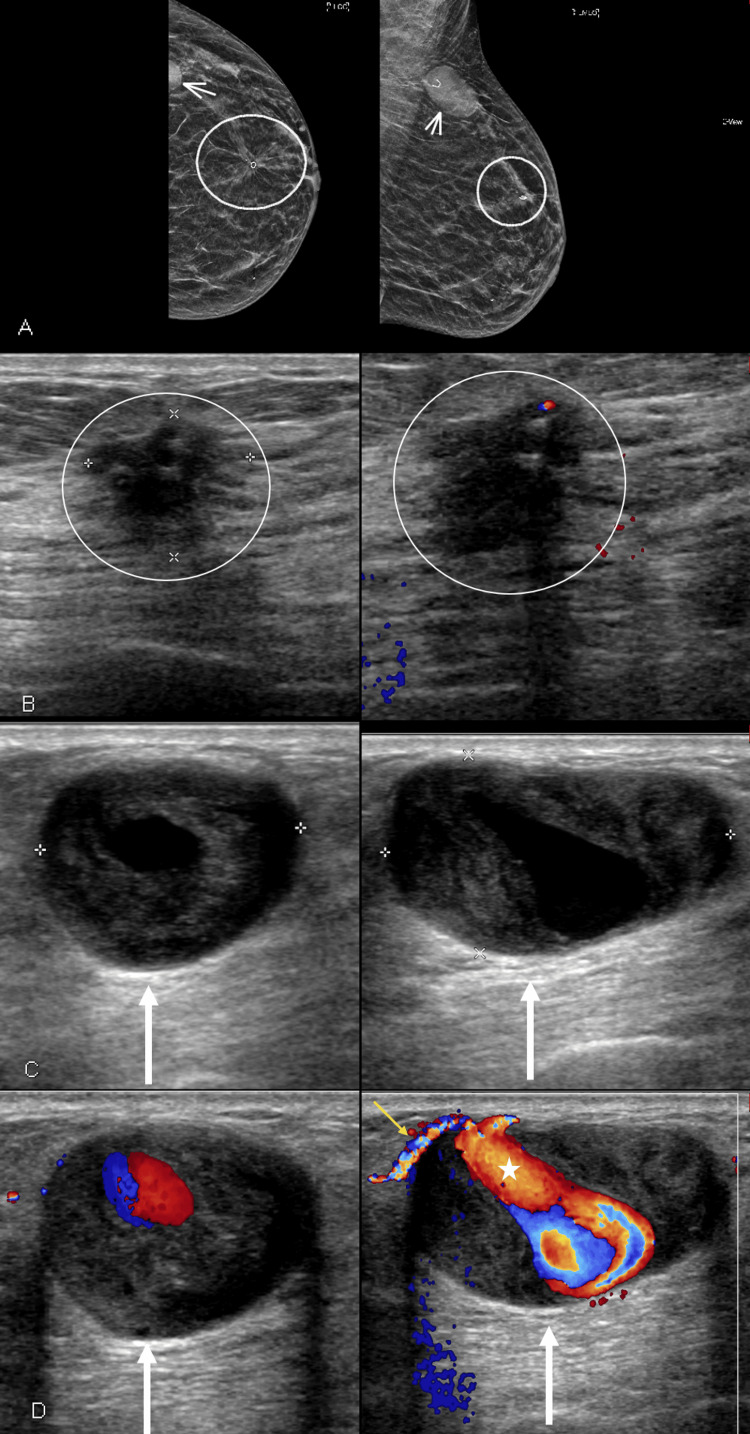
Diagnostic imaging performed five months after the ultrasound guided biopsy A. Mammogram demonstrates a significant decrease in the size of the upper breast mass (circle), but a significant interval increase in the size of the axillary tail asymmetry (arrow) compared to the prior mammogram. B. Ultrasound demonstrates the spiculated mass (circles), representing biopsy-proven infiltrating ductal carcinoma, has decreased in size compared to prior ultrasound. C. Ultrasound demonstrates a significant increase in the size of the previously biopsied lymph node (arrows). D. Doppler flow imaging of the lymph node demonstrates a “ying-yang” sign typical of pseudoaneurysm (arrows). Pseudoaneurysm neck (white star) and feeding vessel (small yellow arrow) are also noted.
